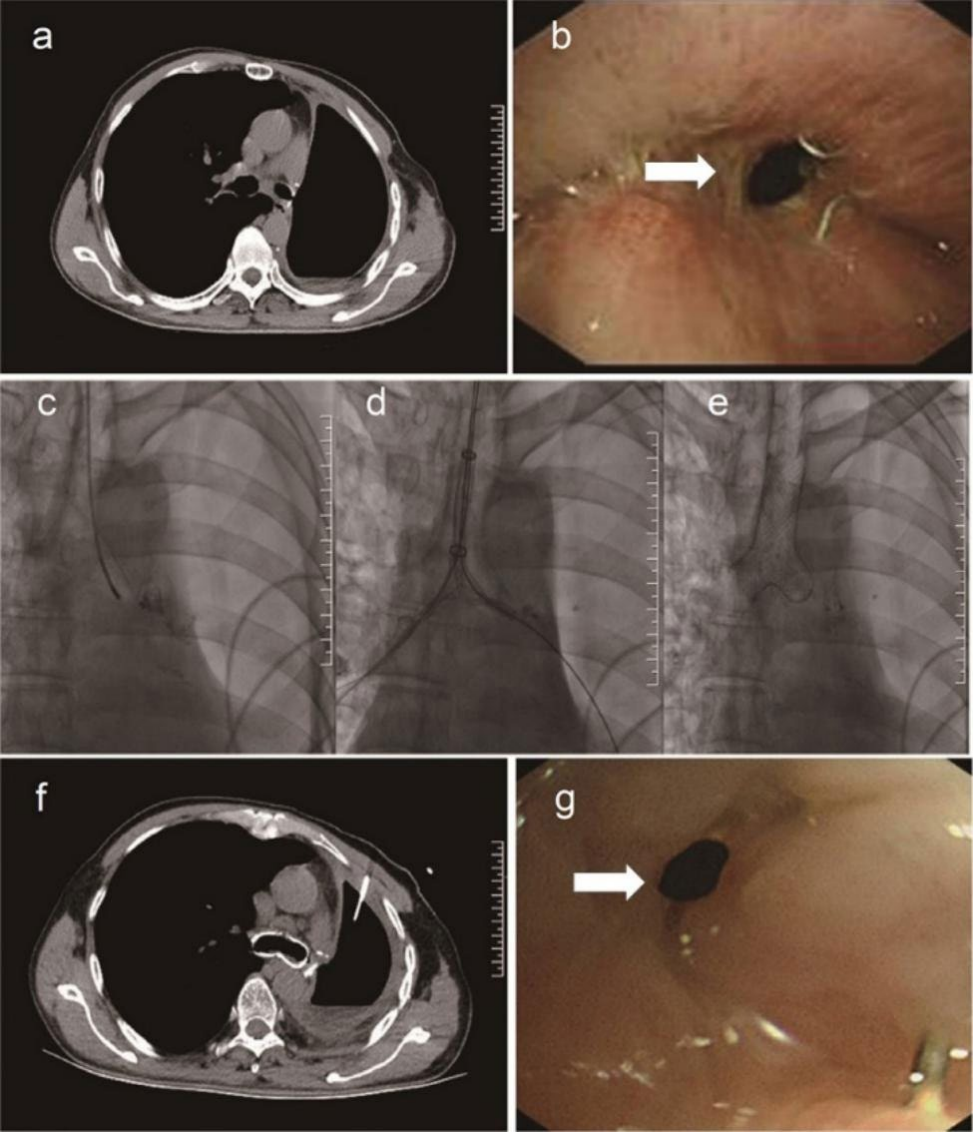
BPF patient failed to be cured by a Y-shaped covered metallic stent with one dead-end branch. CT (a) and bronchoscopy (b) showing the BPF (arrow) after pulmonary lobectomy. Bronchogram demonstrates the formation of BPF (c). The deployment of a Y-shaped covered metallic stent with one dead-end branch under the guidance of fluoroscopy (d). After stent placement, the BPF was occluded by the dead-end branch of the Y-shaped covered metallic stent (e). CT showed that the dead-end branch fit the residual bronchus (f). Three months post-stenting, the stent was removed due to granulation of the end of the stent, but the fistula (arrow) was not cured (g)
